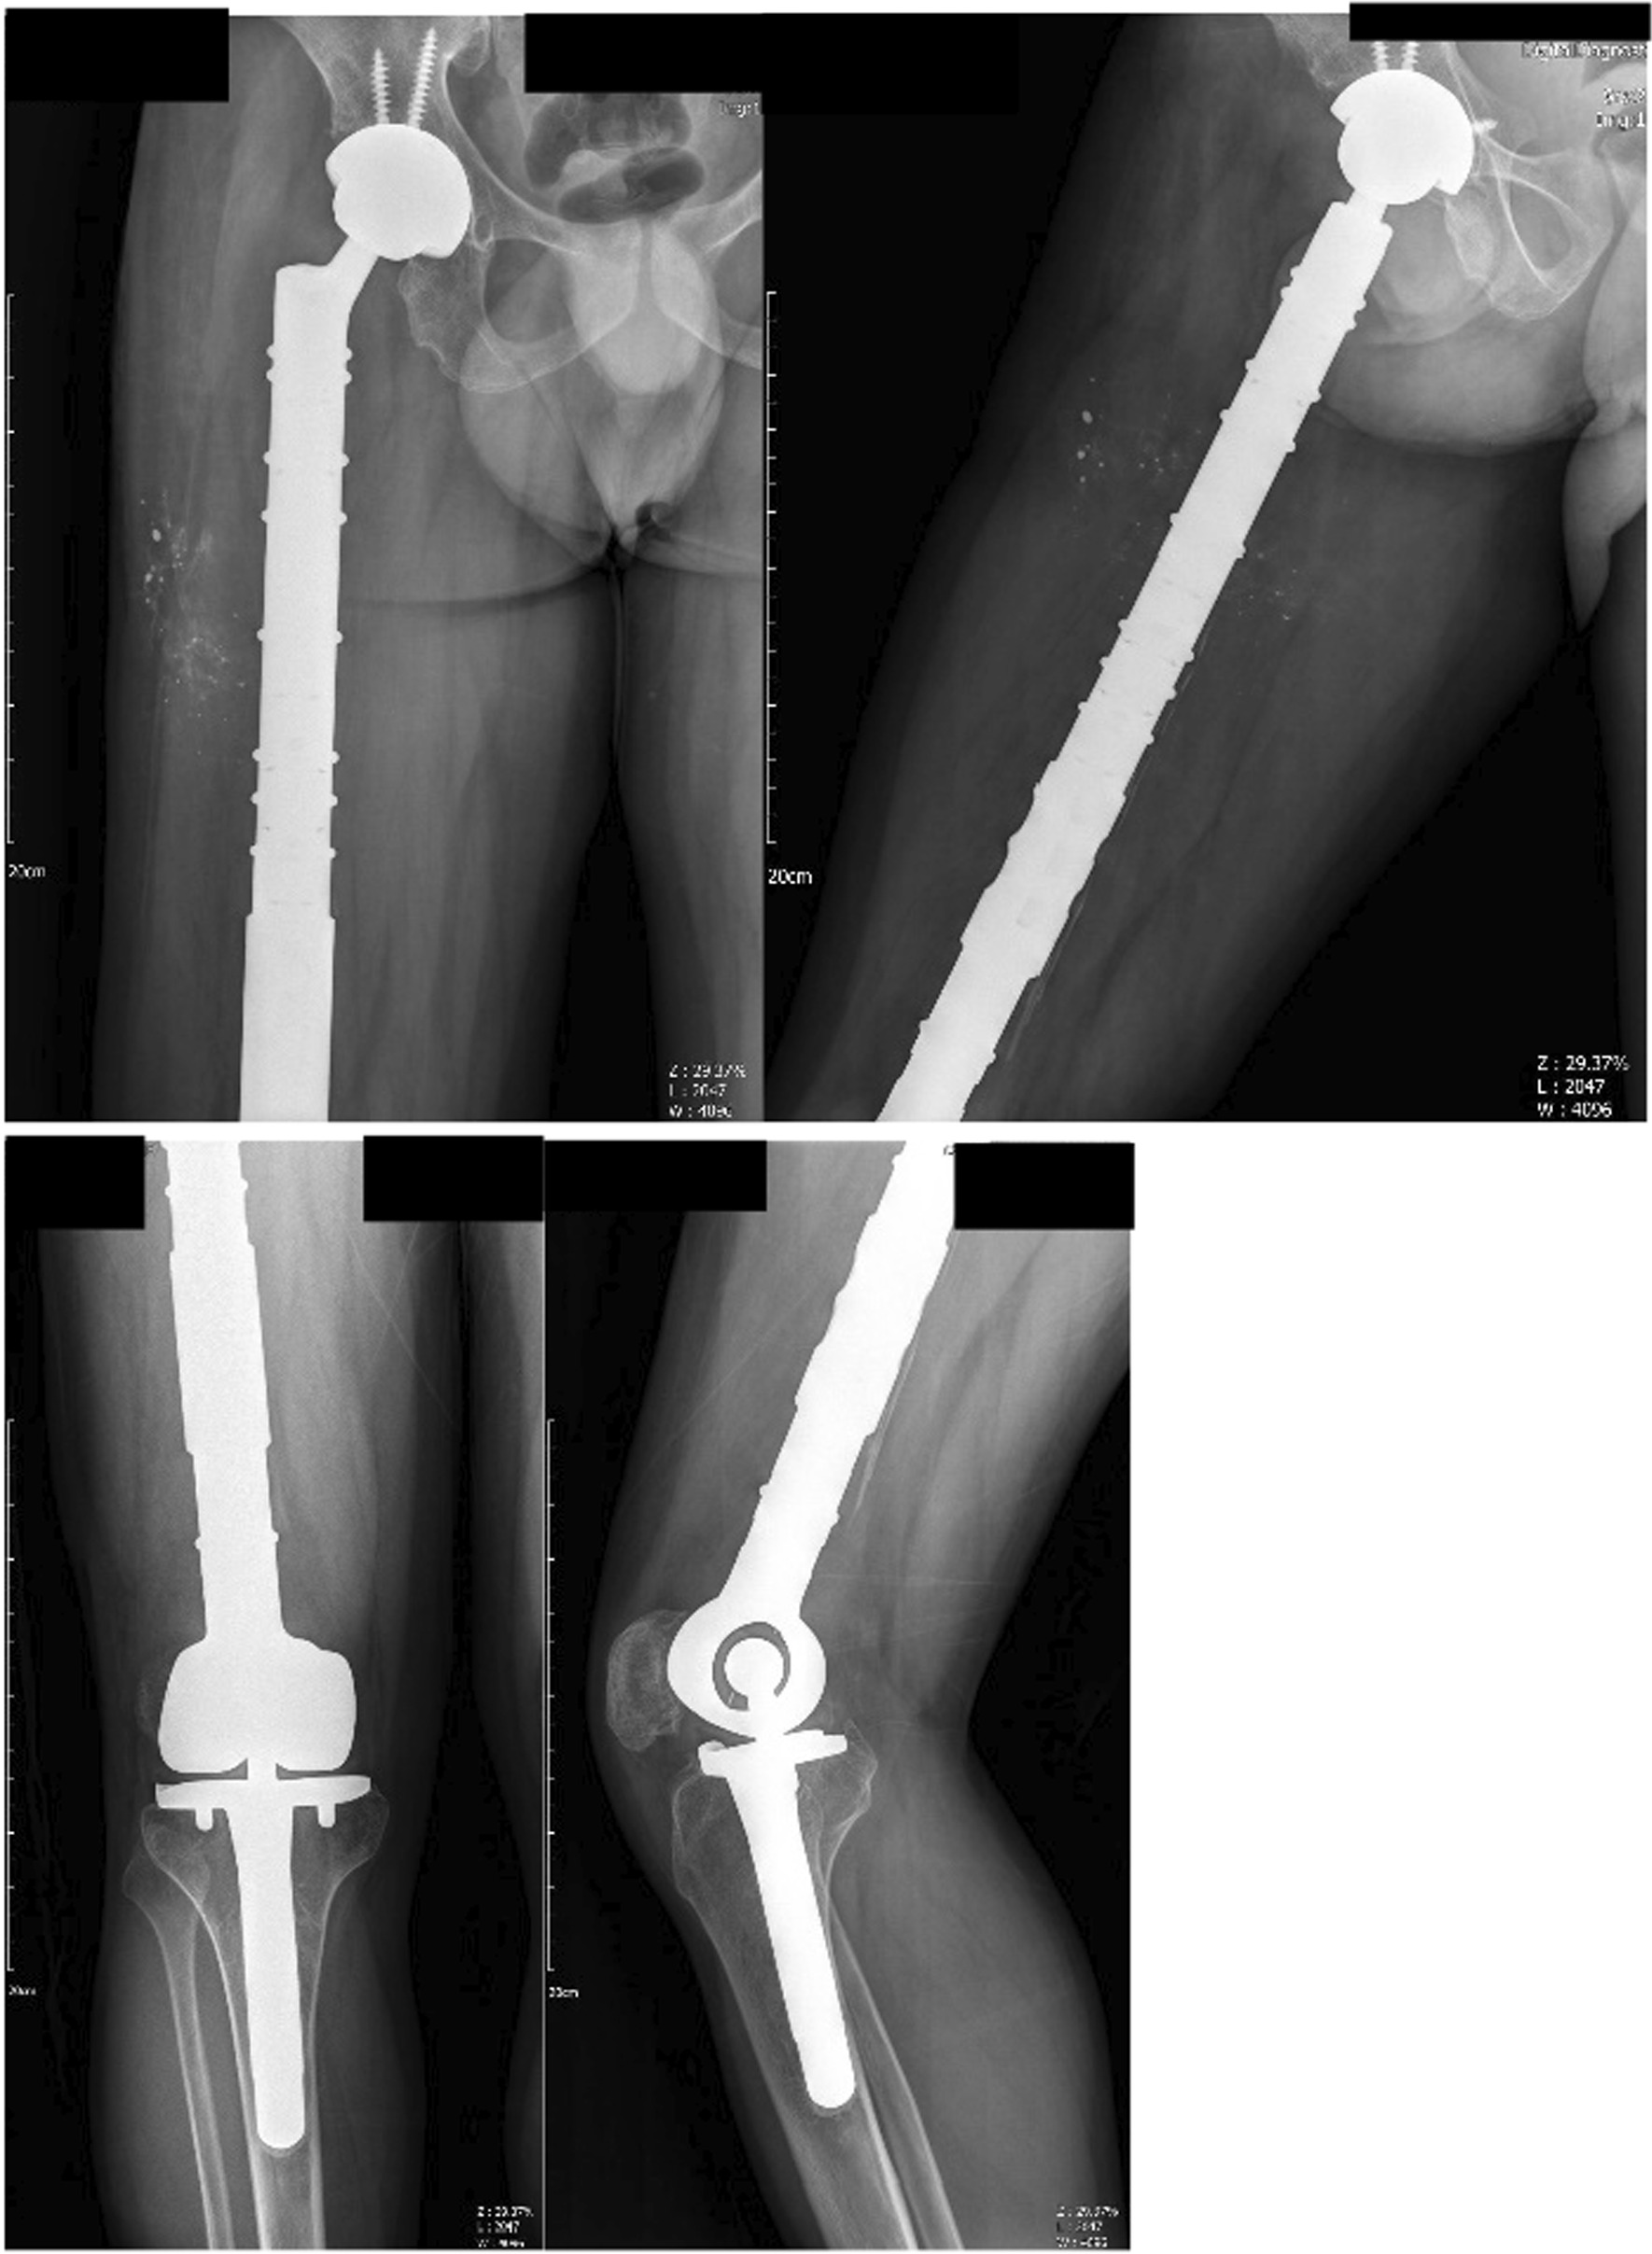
X-ray images 5 years postoperative
